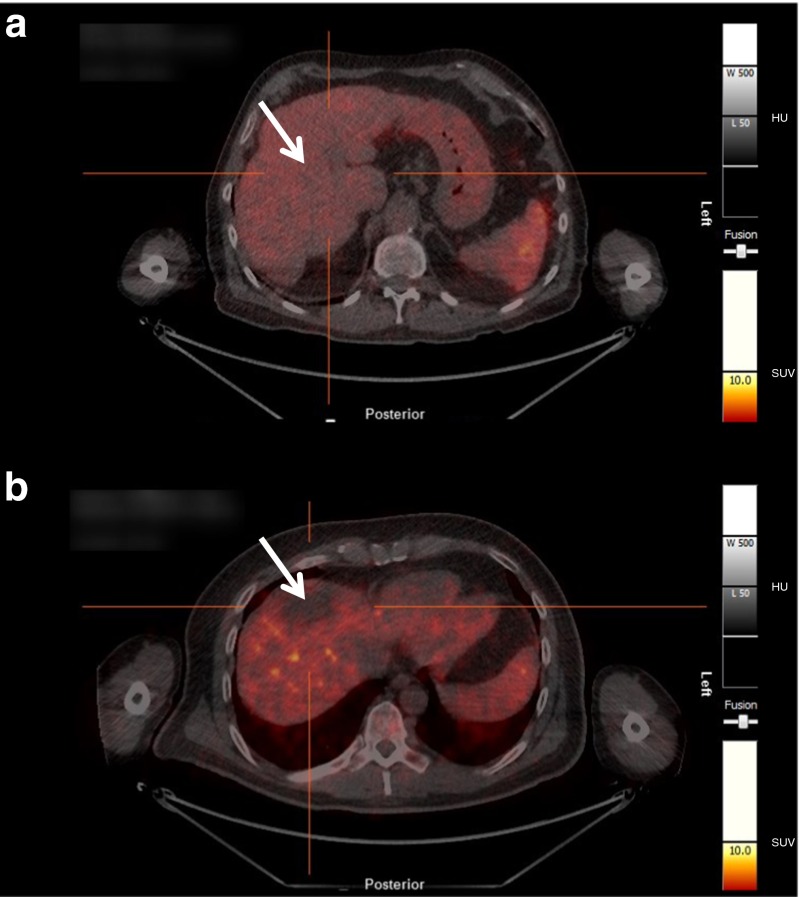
Transaxial PET/CT images of the tumor region. a Patient 7: Tracer uptake in the tumor is comparable with background radioactivity of the liver. b Patient 4: A deficit uptake is found in the lesion compared to the background radioactivity of the liver. *Arrows* indicate the position of the lesions
